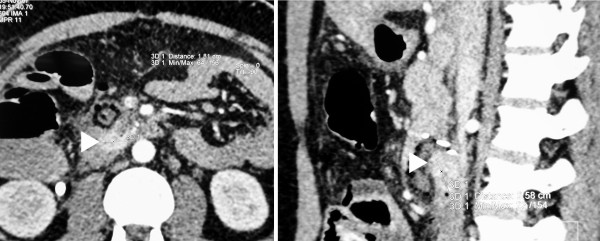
Computed tomography. The computed tomography showed an enhancing tumor of the duodenal wall (arrowheads) with a maximal diameter of 2.5 cm. No metastases were visualized.
